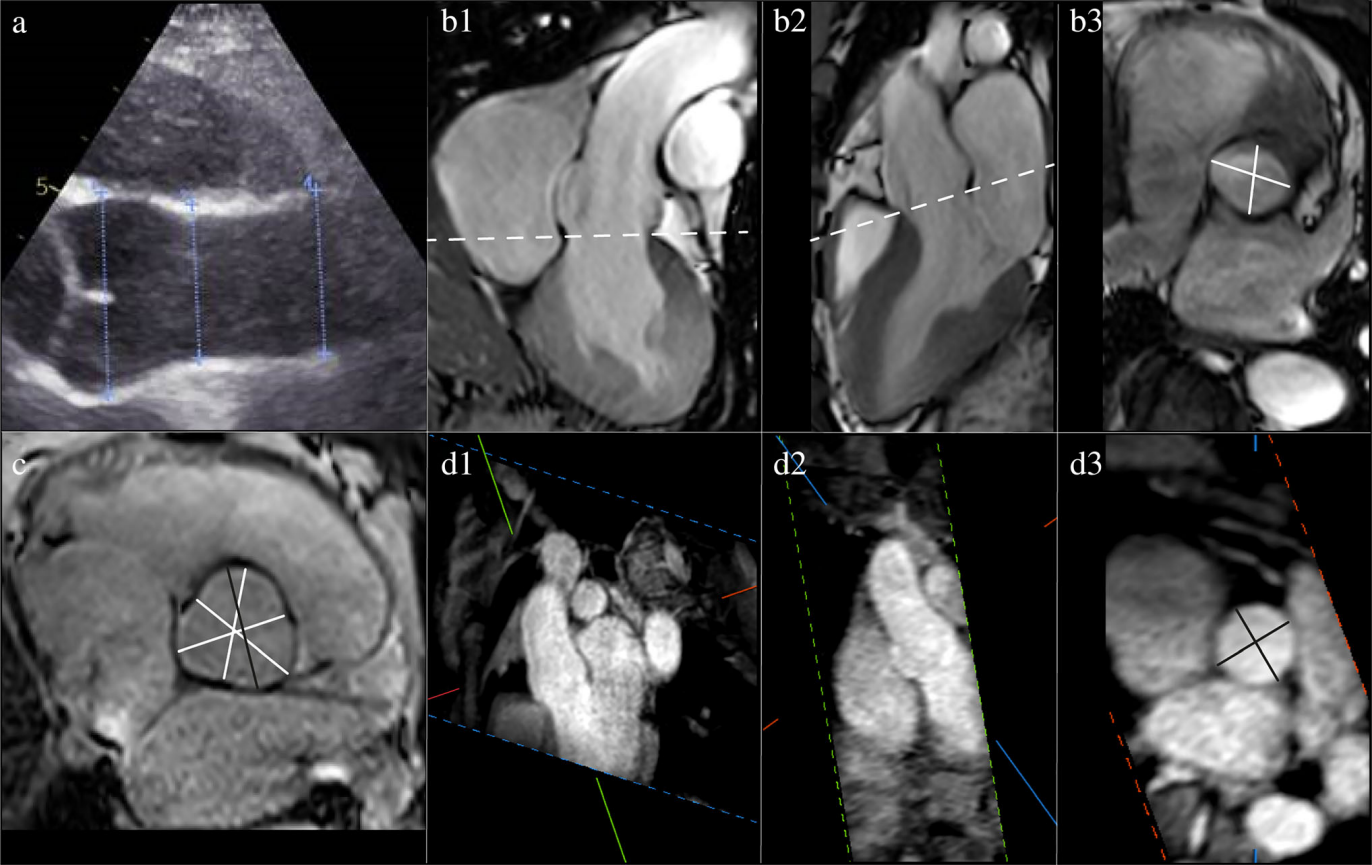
Echocardiographic and MRI measurements of a healthy proximal aorta. (a) Echocardiographic end‐diastolic leading edge‐to‐leading edge measurement of the sinus of Valsalva, sinotubular junction, and ascending aorta. (b) Coronal (b1) and sagittal (b2) planning views for double‐oblique (b3) MRI inner edge‐to‐inner edge systolic measurement of the annulus. (c) MRI average cusp‐to‐commissure and largest cusp‐to‐cusp measurement of the sinus during end‐diastole (with closed aortic valves). (d) MRA planning views (d1) and (d2) for double‐oblique (d3) MRI inner edge‐to‐inner edge diastolic measurement of the sinotubular junction.
